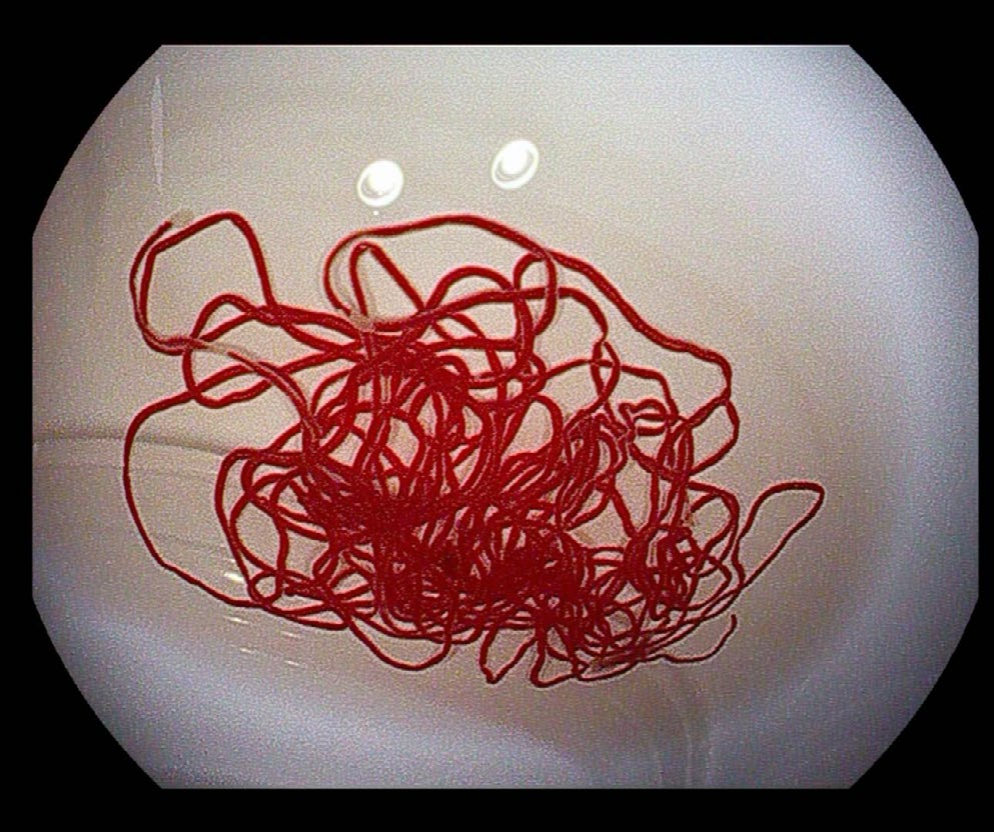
Strips of tissue visible by ultrasonic endoscopic fine‐needle.
